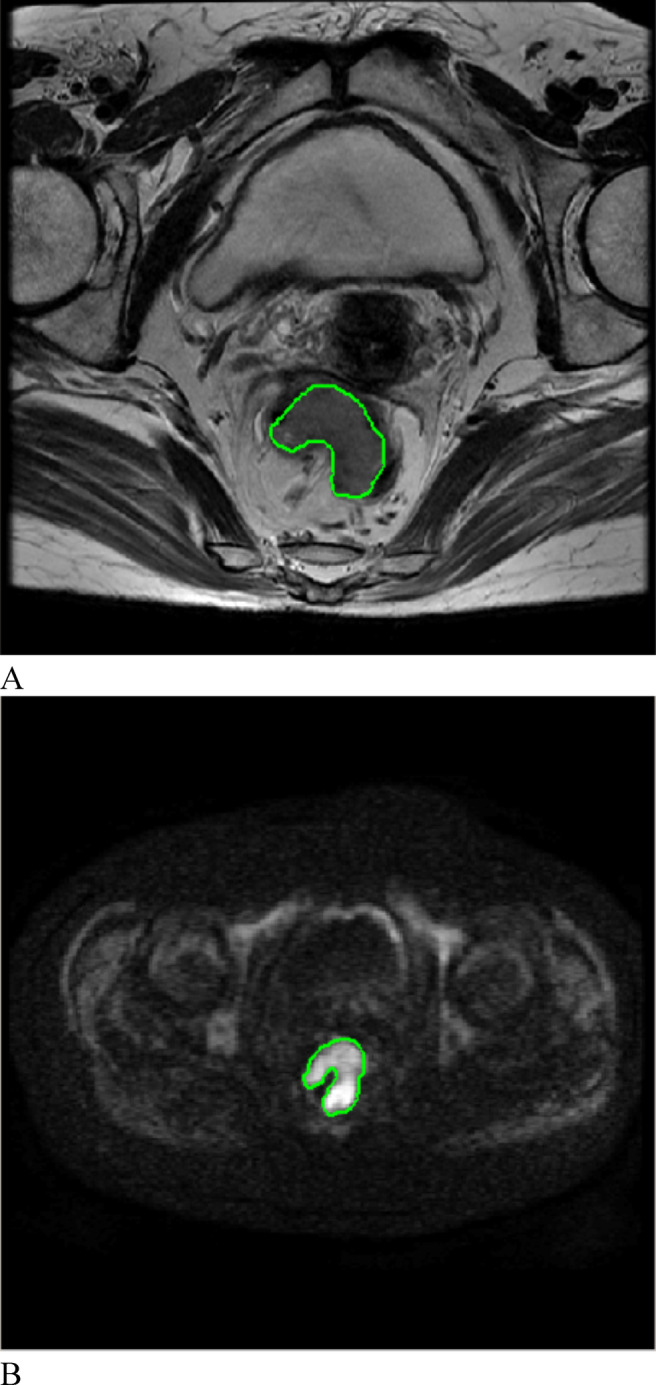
On oblique axial T_2_WI and DWI, the ROI was manually delineated layer by layer along the edge of the rectal cancer lesion. (A) T_2_-weighted imaging; (B) diffusion-weighted imaging.
